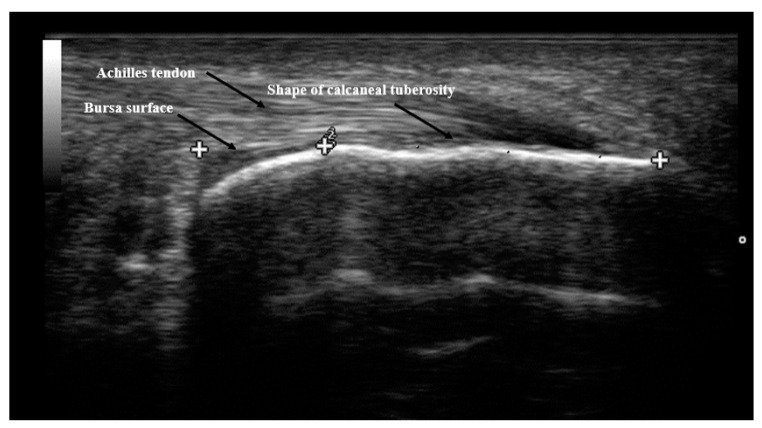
Ultrasonographic image of flat shape of the calcaneal tuberosity.
